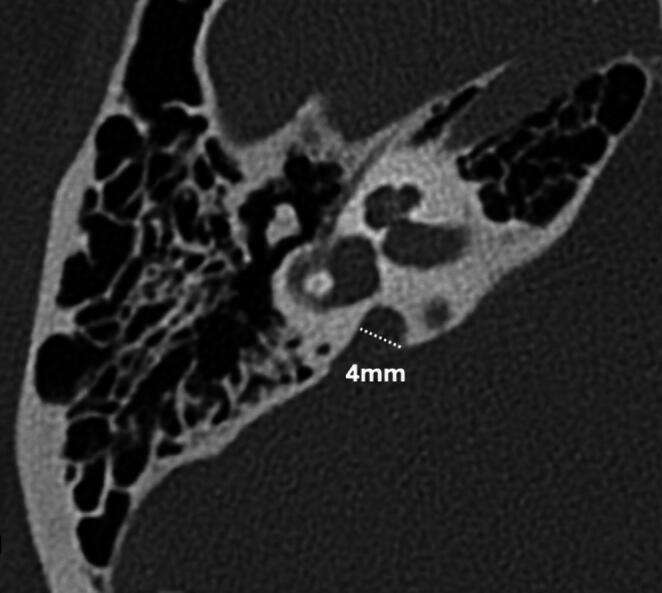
Enlarged vestibular aqueduct (EVA). Axial CT showing an enlarged vestibular aqueduct with opercular diameter > 1.9 mm. EVA is associated with an increased risk of cerebrospinal fluid gusher during cochlear implantation
